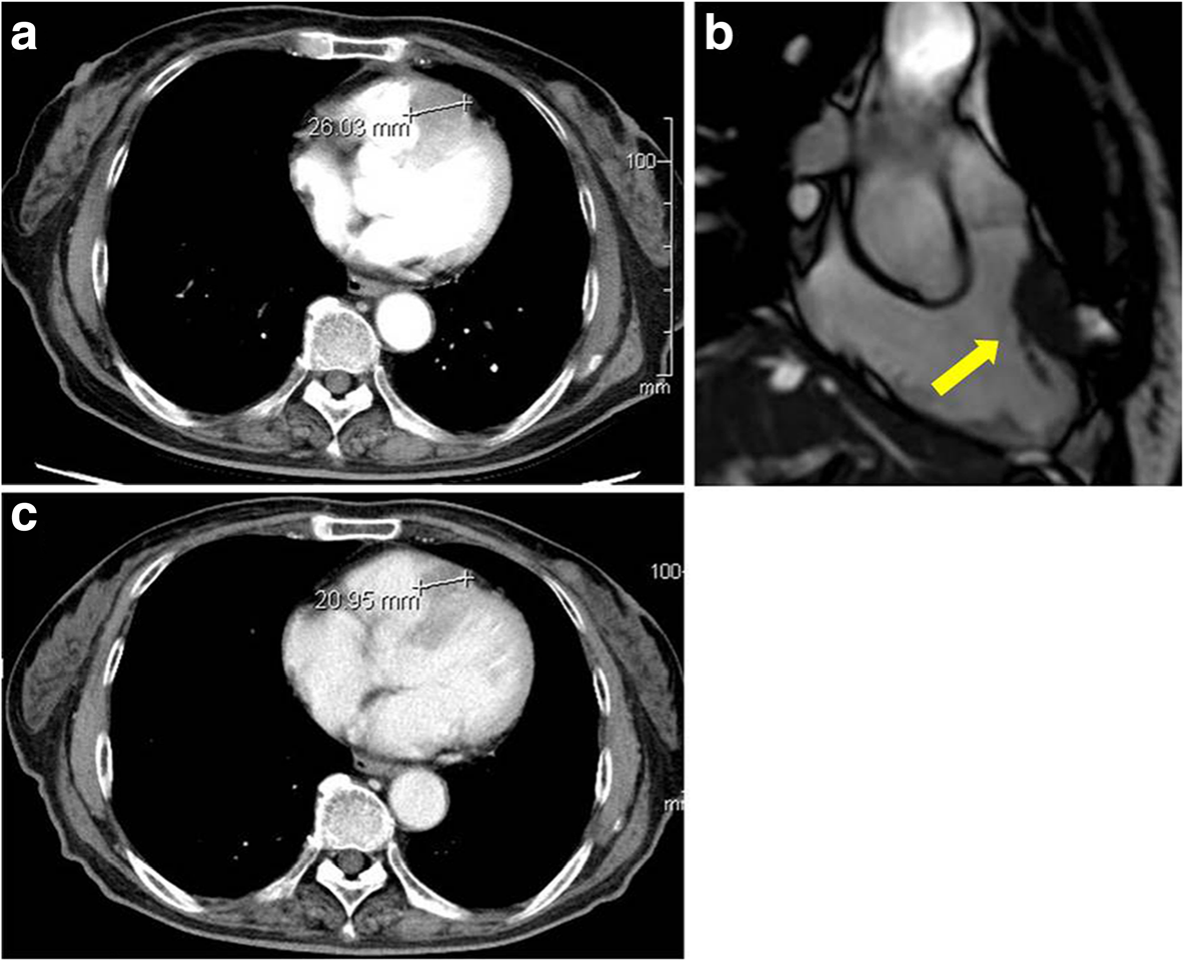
Therapeutic response. A follow-up computed tomography (CT) scan showing the smaller cardiac tumor after 3 months and 4 courses of chemotherapy] (a). A follow-up magnetic resonance imaging (MRI) after 8 courses of chemotherapy also shows a sustained reduction in the tumor size (b). CT after 10 months and 12 courses of chemotherapy (c)
